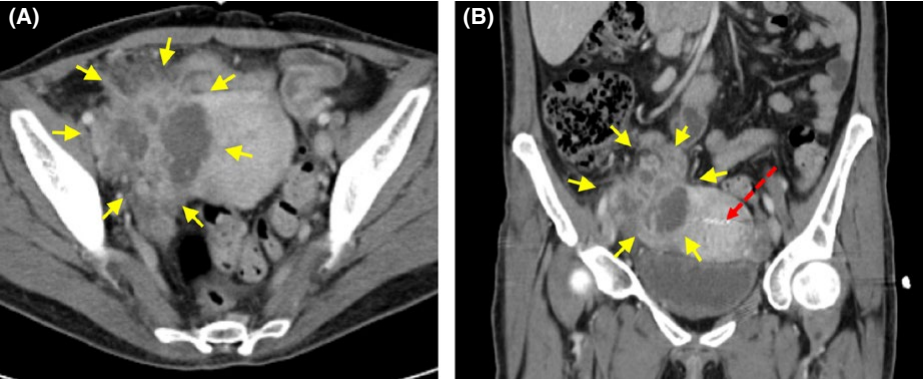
Computed tomography (CT) scan of the abdomen and pelvis of a female presenting with acute abdominal pain and found to have right lower quadrant tenderness with guarding, cervical motion tenderness, leukocytosis (white blood cell count of 15.9 × 10^9^ cells/L), and vital signs within normal limits. Imaging demonstrated heterogeneous solid and cystic soft tissue mass in the right adnexal region with surrounding enteric inflammatory changes, suggestive of appendiceal abscess vs tubo‐ovarian abscess. Overall area of involvement measures 9 × 7 × 6 cm (yellow arrows). A retained intrauterine device (red arrow) of 17 years was also visualized. A, Axial view. B, Coronal view
